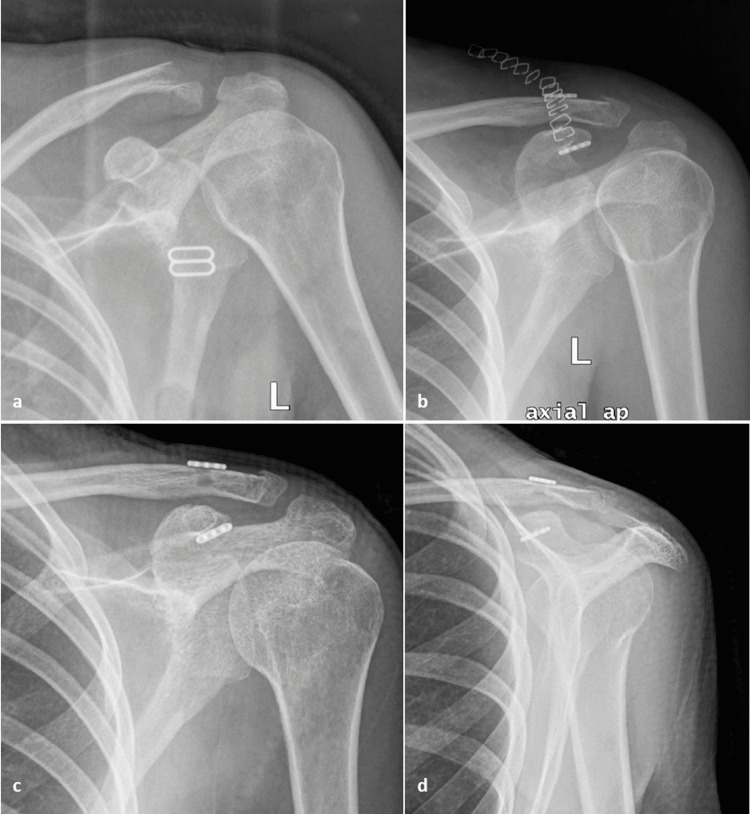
Radiological evaluation of patient 1. (a) Preoperative anteroposterior radiograph showing an extralateral fracture of the distal clavicle. (b) Excellent postoperative reduction using a closed-loop double button system. (c, d) Last follow-up radiographs (anteroposterior and Alexander view), 12 months postoperatively, showing maintenance of reduction and no signs of osteolysis or loosening.
